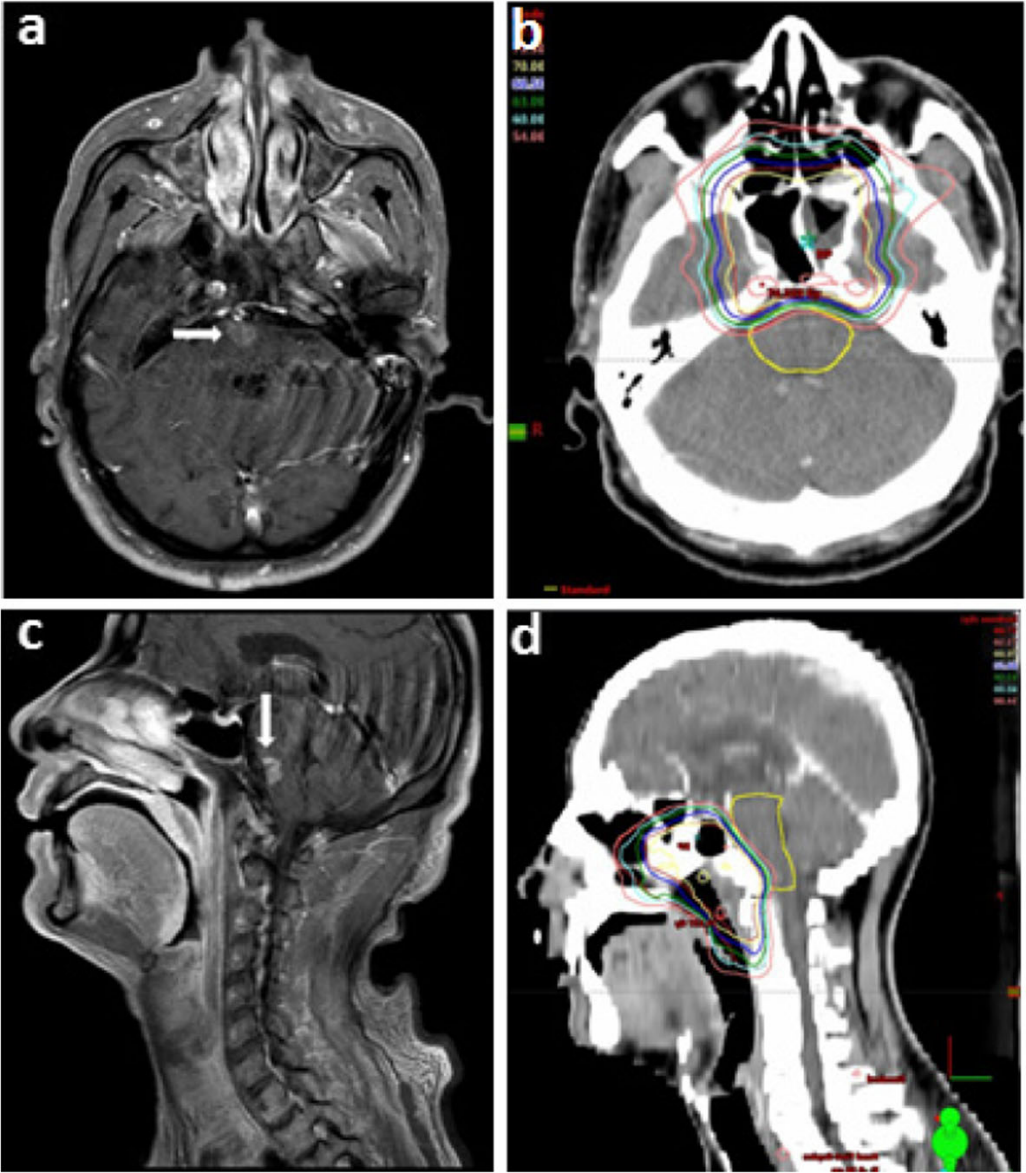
Necrosis nidus within the brainstem and the corresponding dose distribution. Contrast-enhanced lesion (white arrow) in the axial (a) and sagittal (c) view on post-contrast T1-weighted MRI images with a 53-year-old NPC patient (the 5th patient in Table 1). Corresponding isodose lines are shown in b and d, respectively
